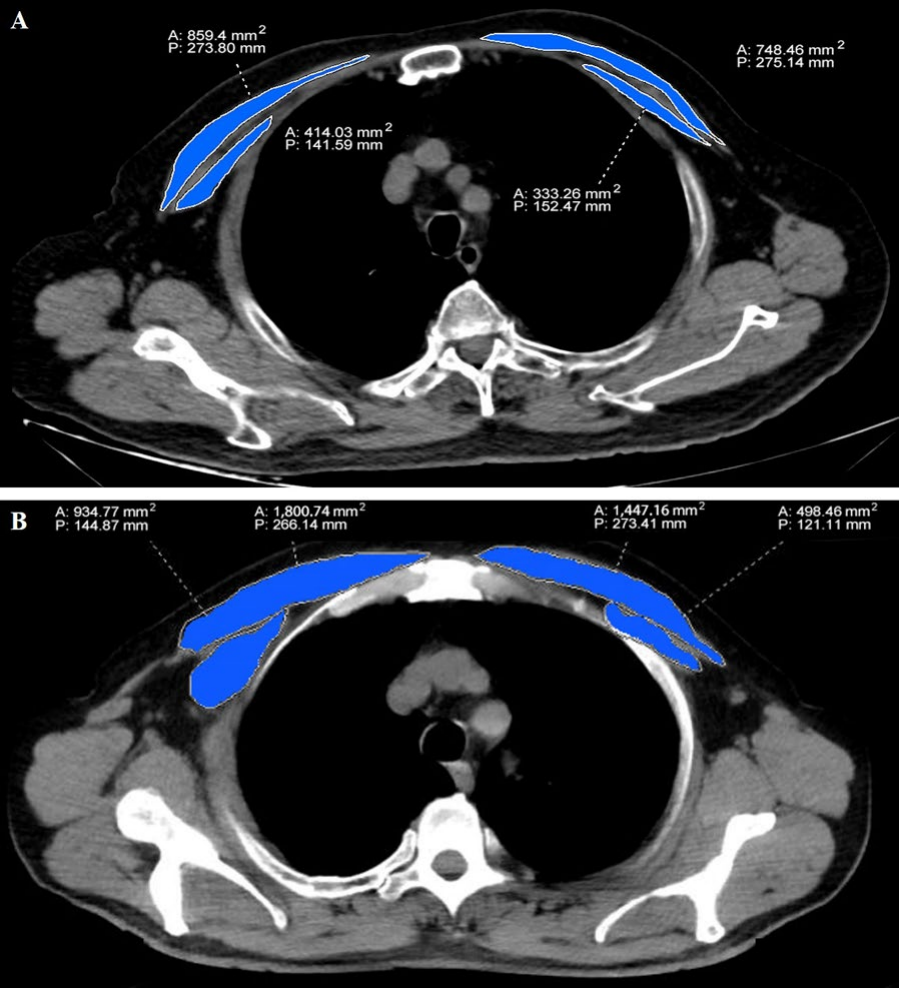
The pectoralis muscle area (PMA) measurement on chest CT image. Bilateral pectoralis muscles are colored blue (pectoralis major and minor muscles). a A 67-year-old male patient with COVID-19. b A 72-year-old male patient with COVID-19. A, Area, P, Perimeter
